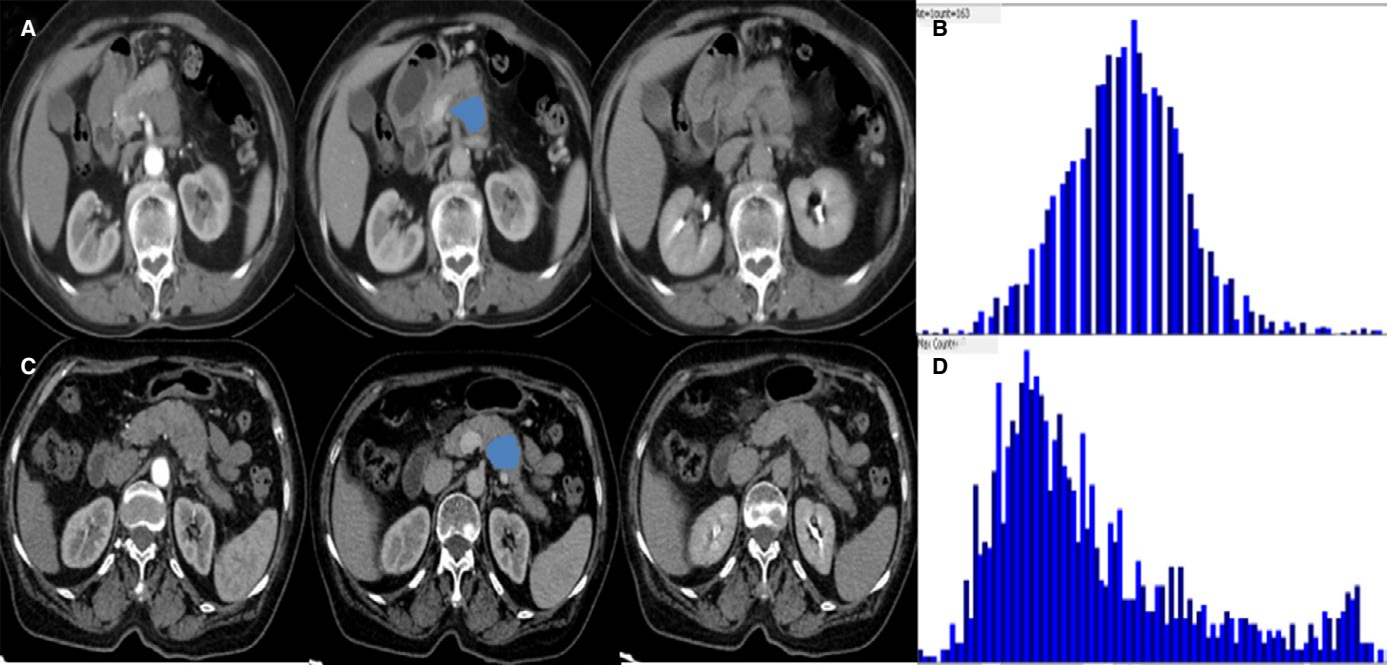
Examples of atypical pancreatic neuroendocrine tumors (pNETs) and pancreatic ductal adenocarcinomas (PDACs) using three‐phase CT scan. A, The arterial phase showed a hyporvascular lesion in pancreatic head. B, The image gray‐level histogram showed a skewness value of 0.31 of pixel‐wise attenuation on portal phase. C, The arterial phase showed a hypovascular lesion in pancreatic body. D, The image gray‐level histogram showed a skewness of 1.1 of pixel‐wise attenuation on portal phase
